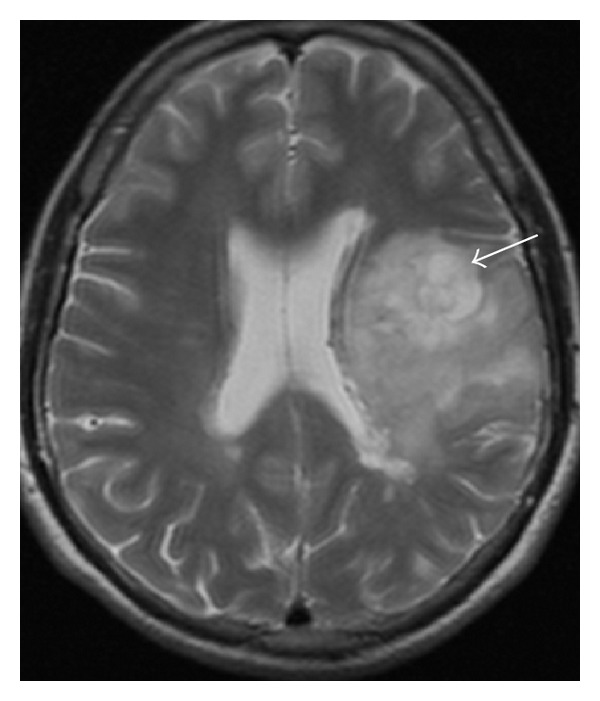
Cerebral MRI (2003): large left frontal lobe lesion (arrow) suggesting a tumefactive demyelinating lesion.
